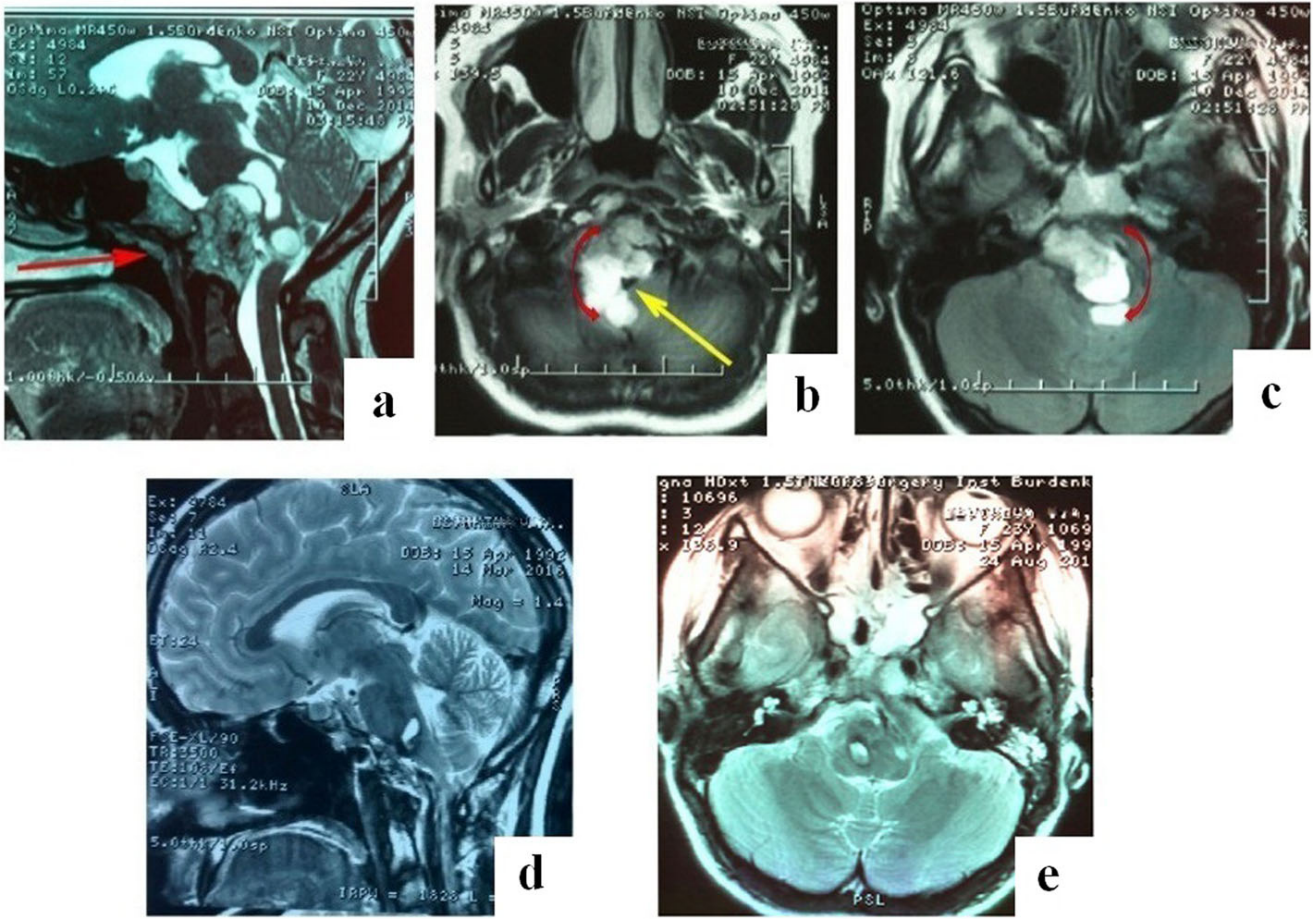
Case 2. Female patient, 22 years old, with giant chordoma of the skull base, with severe compression of the medulla oblongata and midbrain. a–c Preoperative MRI images: a Straight red arrow indicates the direction of transnasal approach. b, c Curved red arrows indicate the direction of approach to the posterior portion of the tumor around the brain stem structures. b Yellow arrow indicates basilar artery location. d, e MRI images 12 months after surgery, subtotal tumor resection
